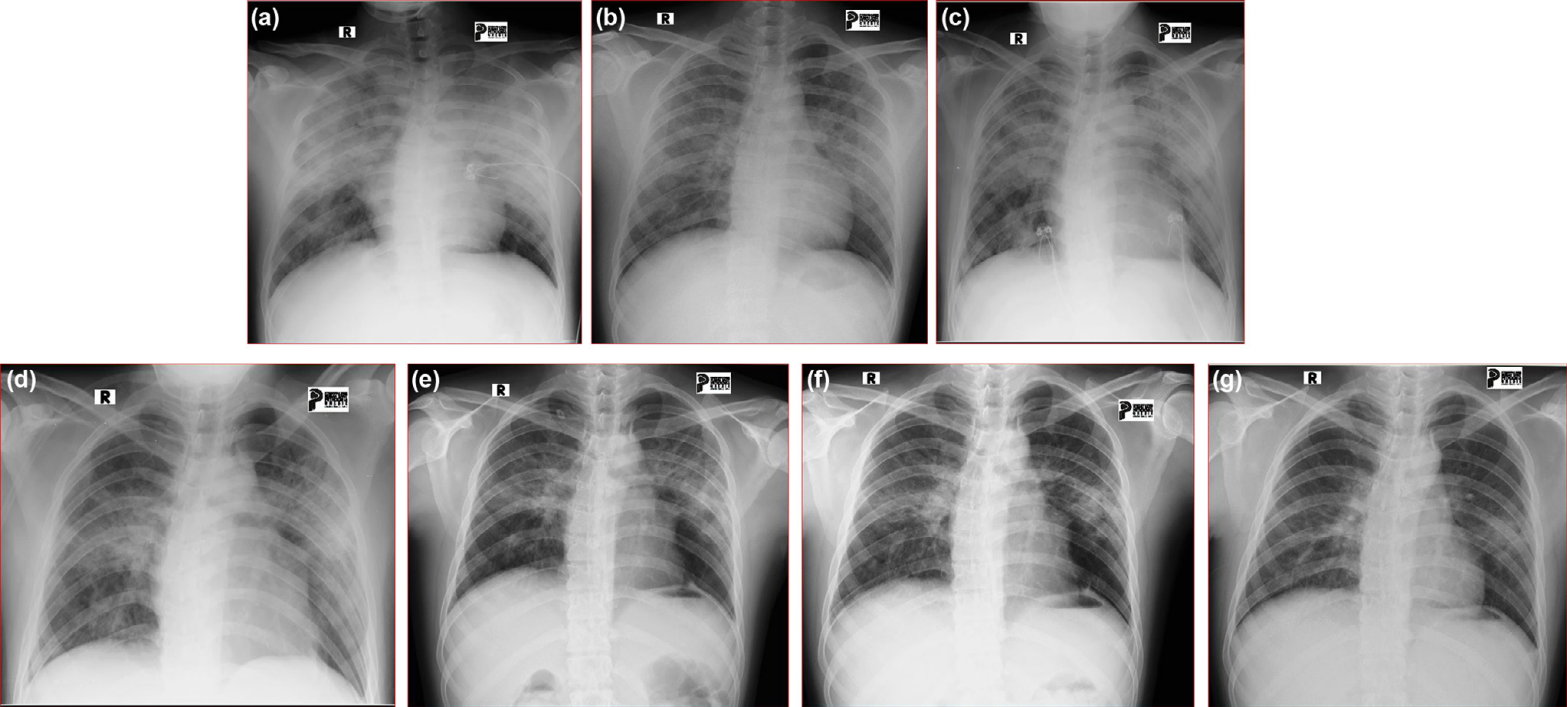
Serial chest X-ray on hospitalization (A. Day 1; B. Day 4; C. Day 7; D. Day 10; E. Day 13; F. Day 18) and discharged on Day 39.
